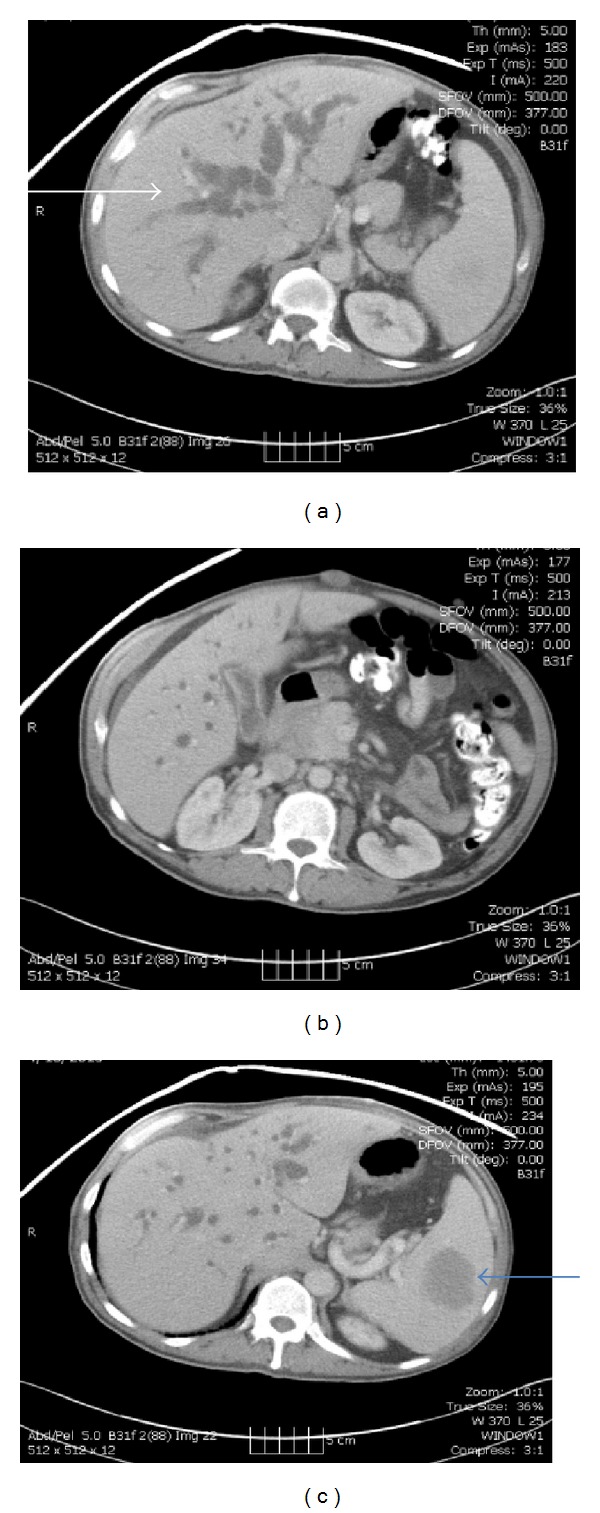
Computed tomography scans of the abdomen showed the porta hepatis mass, markedly dilated intrahepatic bile duct (white arrow), a 5 cm splenic mass (blue arrow), and a retroperitoneal mass.
